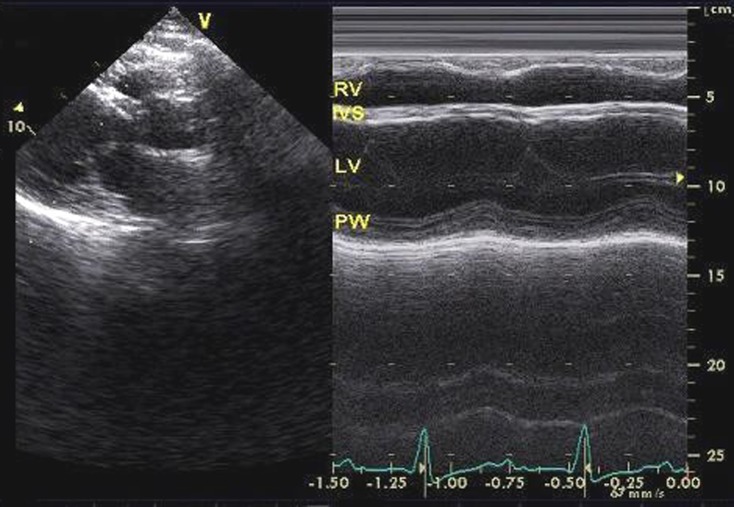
M-mode transthoracic echocardiogram from the parasternal long axis view demonstrating left ventricular dilatation and systolic dysfunction (RV: Right ventricle, IVS: Interventricular septum, LV: Left ventricle, PW: Posterior wall).
